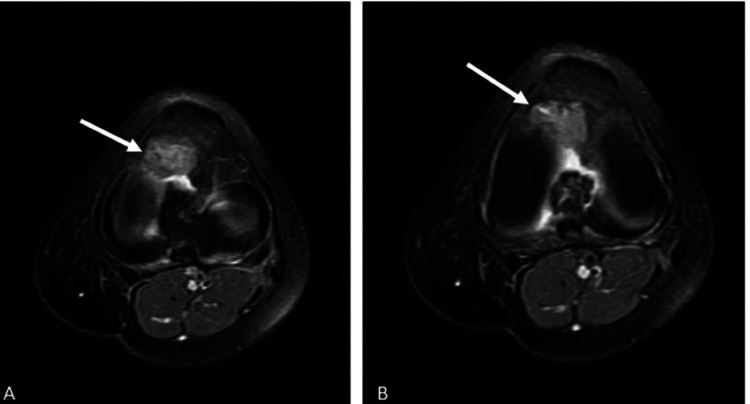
The patient's T2-weighted axial MRI sections. (A) The lesion appears as a hyperintense area in the axial plane, demonstrating its shape and extent relative to surrounding structures. (B) Another axial section further delineating the lesion’s borders and internal characteristics, providing additional details on its spatial orientation The white arrows indicate the lesion MRI: magnetic resonance imaging
